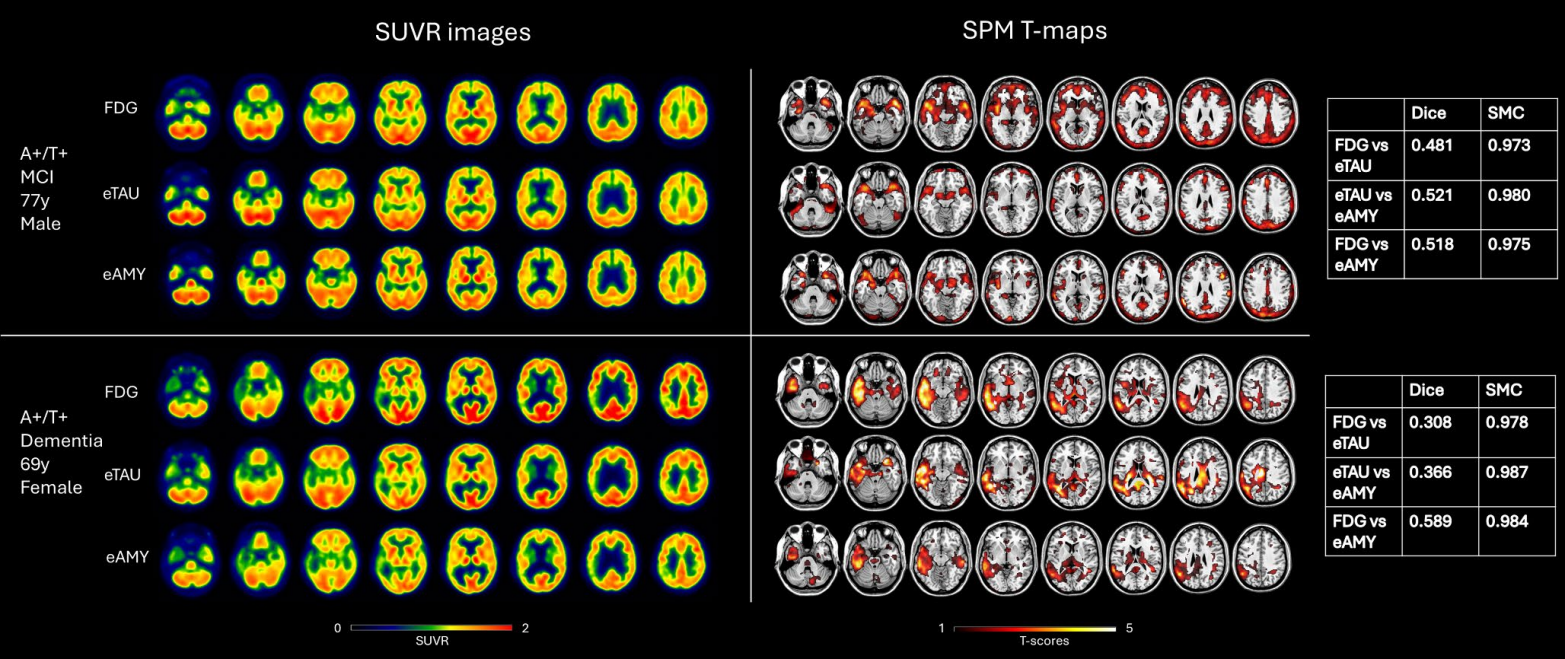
Individual examples of hypometabolic and hypoperfusion standardized uptake distribution images and SPM t-maps. On the left, axial views of representative [^18^F]FDG-PET SUVR, early-phase tau-PET SUVR, early-phase amyloid-PET SUVR images in a patient with prodromal AD (MCI, top row) and in a patient with AD dementia (bottom row). On the right, in the same order, the SPM single-subject T-maps of the same subjects are displayed on the standard Montreal Neurological Institute template. The tables showed the similarity coefficients (Dice and simple matching coefficient (SMC)). A = amyloid; eAMY = early phase amyloid-PET; eTAU = early phase tau-PET; MCI = mild cognitive impairment; T = tau
